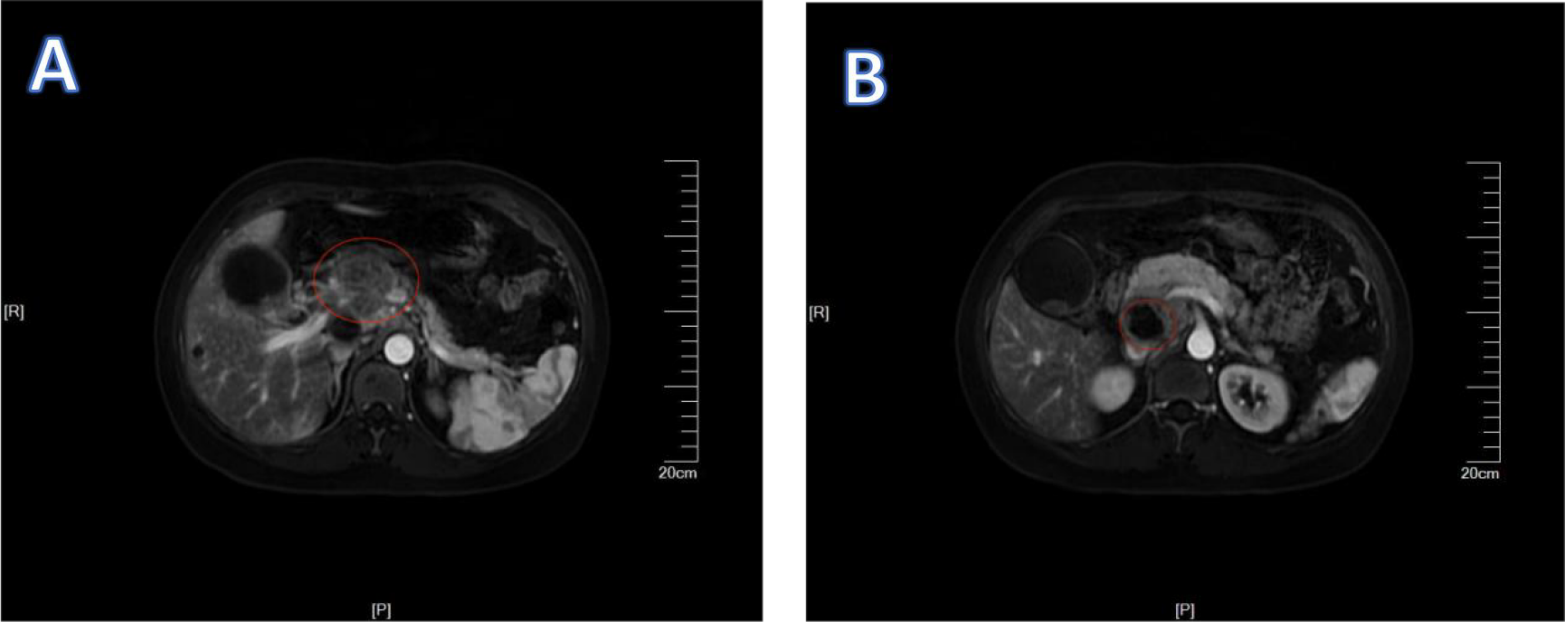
1Patient 1’s MRI results. The red circles in (A, B) indicate swollen lymph nodes.
